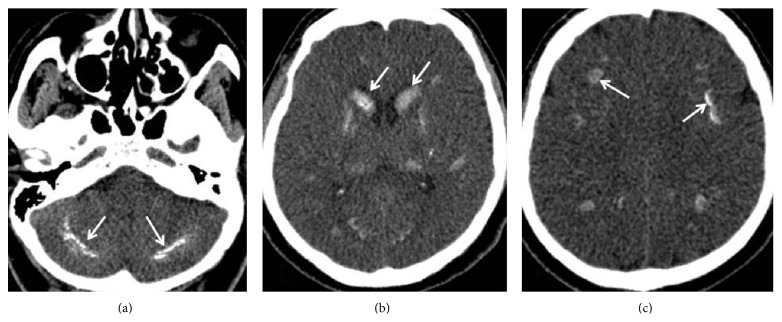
A large number of calcifications are observed in both cerebellar hemispheres (a), basal ganglions (b), and subcortical white matter (c) in axial CT sections (white arrows).
